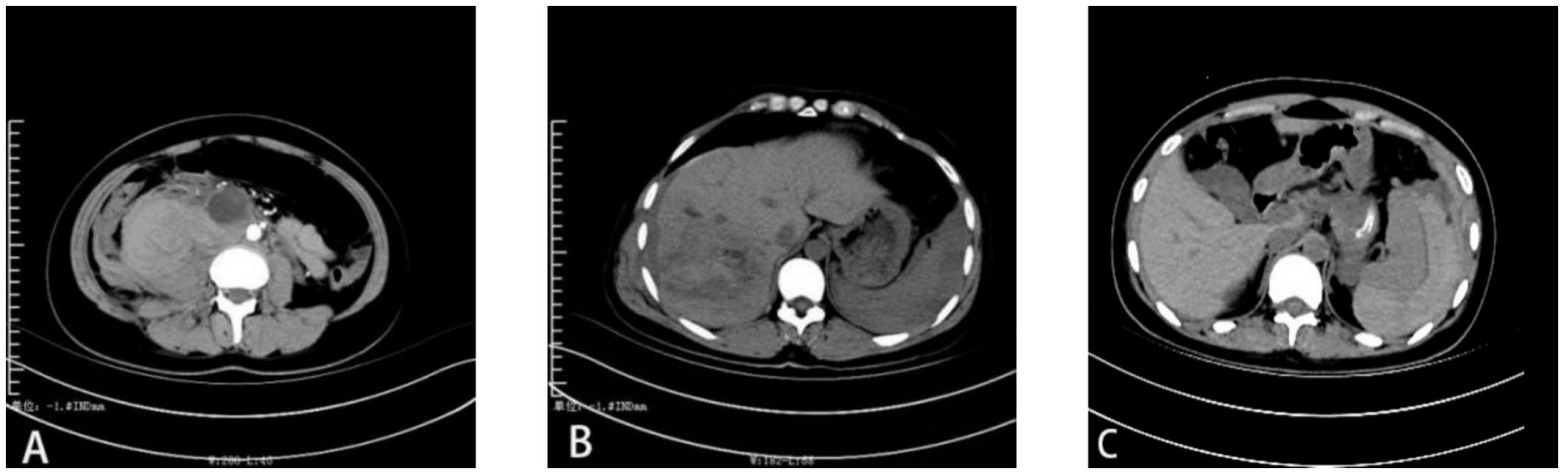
CT images of (A) Retroperitoneal pregnancy of the first patient, (B) hepatic pregnancy of the second patient, and (C) splenic pregnancy of the third patient.
